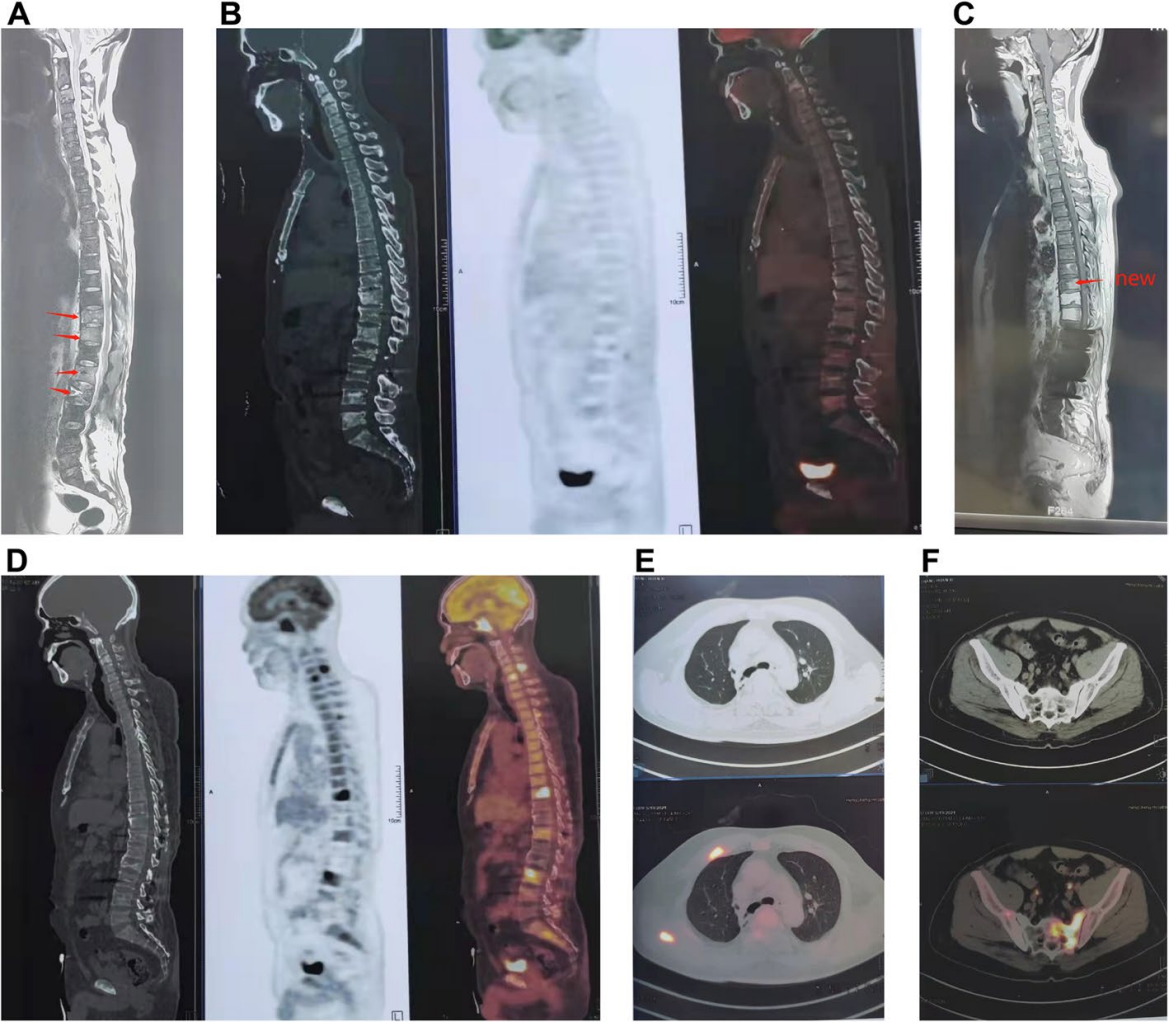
A PET image showing that the hypermetabolic lesions were significantly reduced in numbers compared with those on PET performed in October 2018 after 3 months of anti-fungal treatment (June 2019). B Spinal MR image showing new abnormal lesions at the level of the T9 vertebra. D–E Compared with PET performed in June 2019, PET performed in June 2021 showed that hypermetabolic lesions increased both in size and numbers significantly in the spine, rib, and pelvis. CT, computed tomography; MR, magnetic resonance; PET, positron emission tomography
